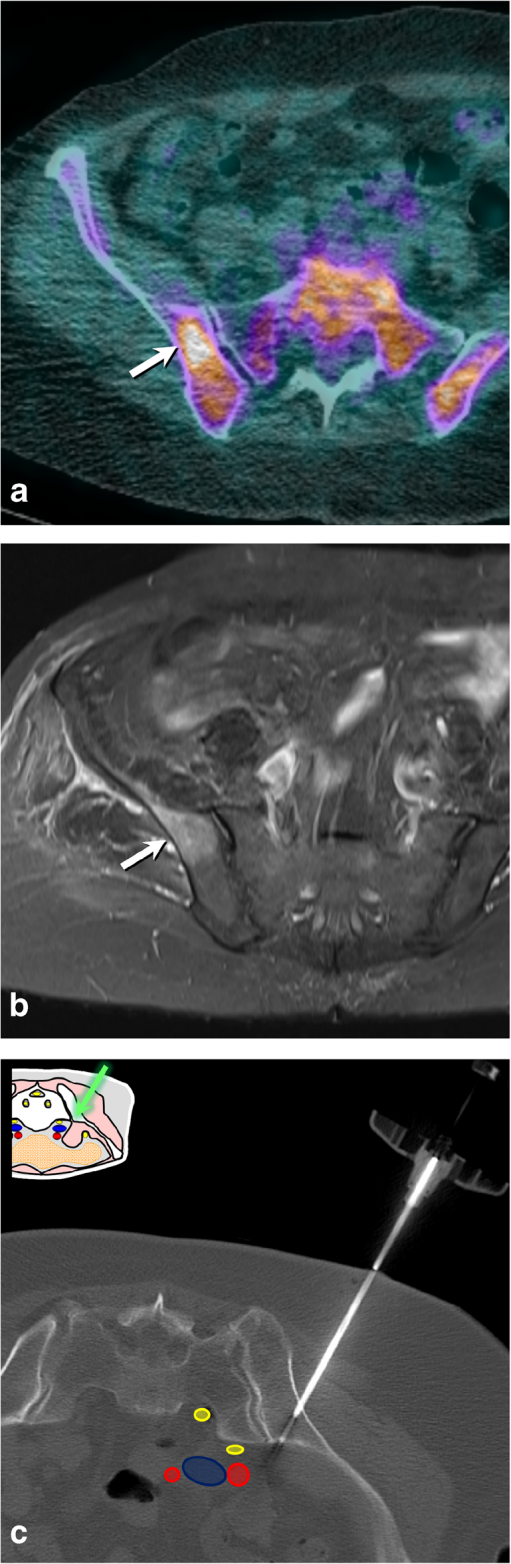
A 77-year-old woman presenting with multiple foci of increased bone metabolism on PET scan and no known primary tumour (a, *arrow*) and abnormal bone marrow signal on MRI (b, *arrow*). The lesions were not visible on CT and a biopsy of the most conspicuous lesion was carefully planned using anatomical landmarks (c). The lesion was targeted through a direct approach and proved to be non-Hodgkin’s lymphoma
